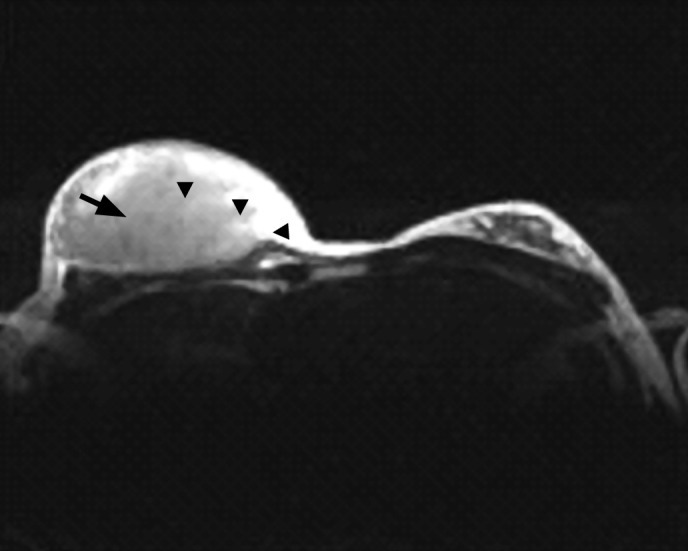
MRI axial T2 weighted image in the same patient shows the irregular mass (arrow heads) with intermediate signal intensity. There is also a dilated duct within the mass (arrow).
